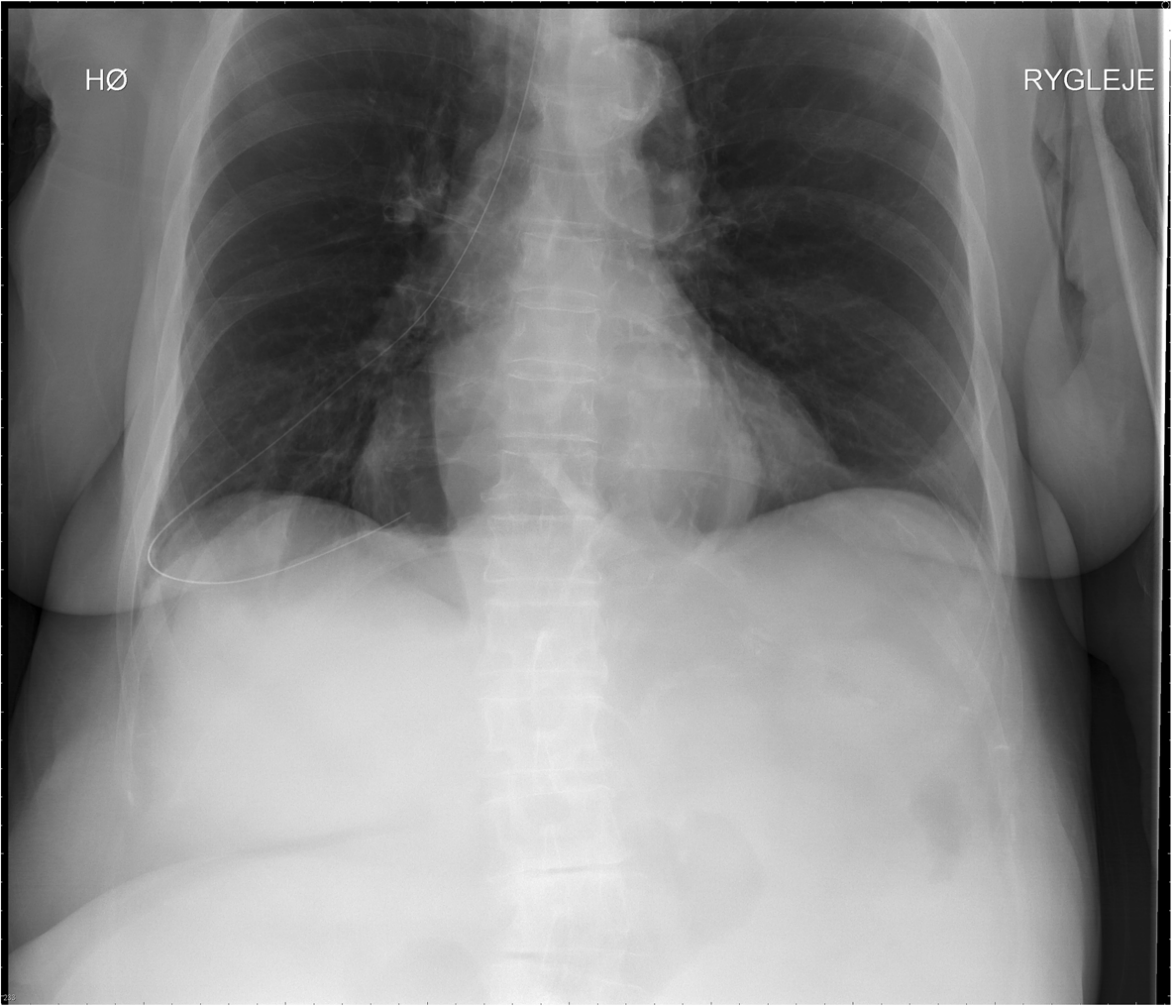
Chest X-ray prior to removal, patient lying down.
